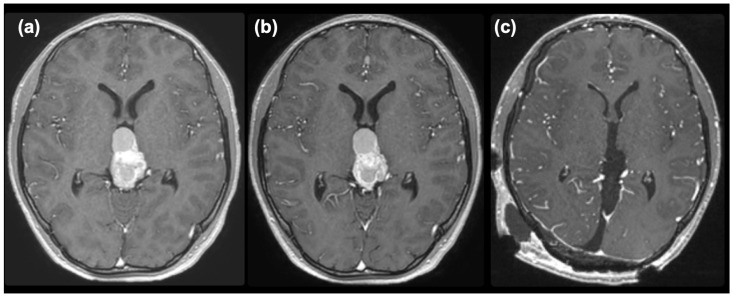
Post-contrast MR images taken after one course (a), two courses (b) of chemotherapy, and after removal (c).
